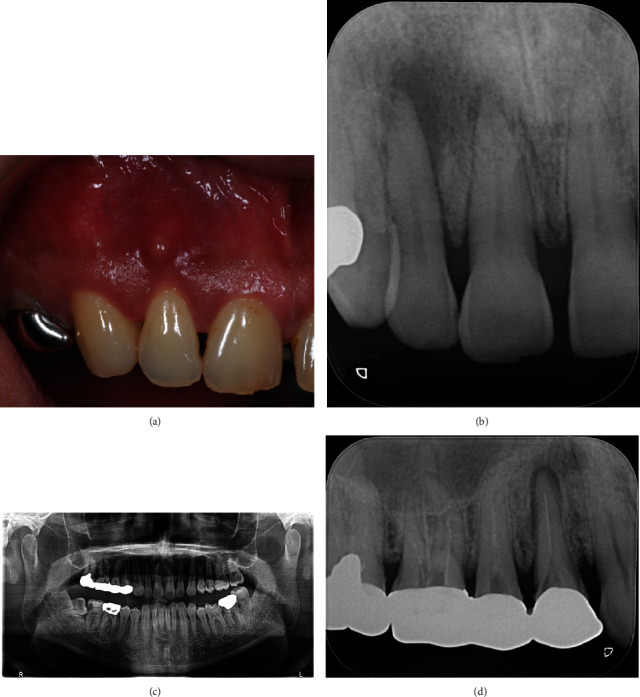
Preoperative photograph and radiographs. Intraoral photographs of the right central and lateral incisor with swelling and spontaneous pain (a). The periodontal pockets were around 1–2 mm circumferentially, and there was no bleeding on probing sites. Intraoral radiographs showed no carious lesions in either incisor, but radiolucent regions were seen around the apexes of the maxillary right central and lateral incisors (b). Both incisors showed normal responses to the dental pulp cold stimulus test. Panoramic radiographs showed numerous apical and carious lesions in the mouth (c). The closest lesion to the site was the maxillary right first premolar. Intraoral periapical radiographs showed apical lesions of the maxillary right first premolar (d).
